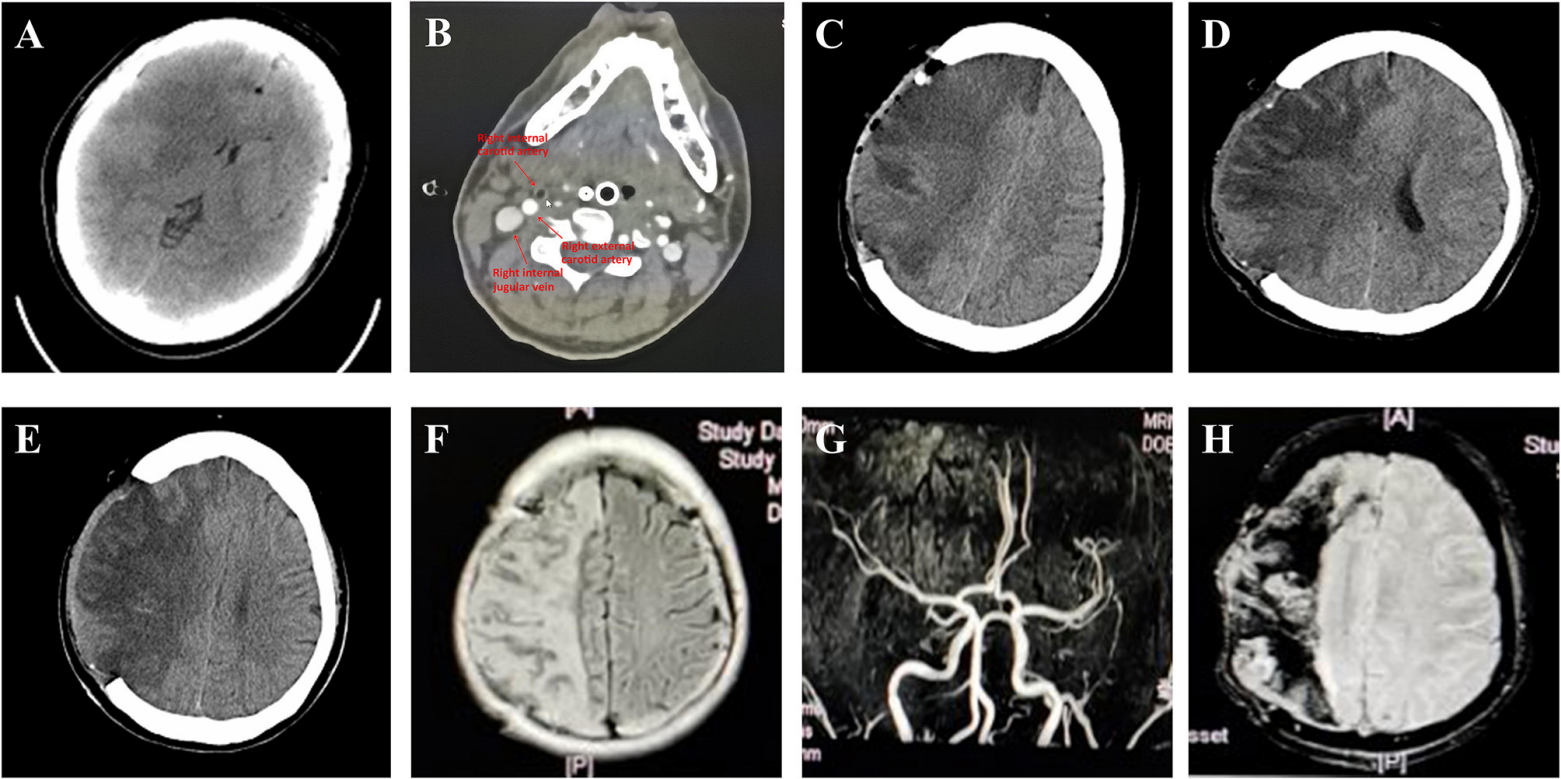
Imaging in different stages after cerebral infarction of this case. (A) Brain CT imaging of the patient shows massive cerebral infarction on the right frontal, temporal, and parietal lobes, and basal ganglia. (B) The neck CTA suggested that the right external carotid artery was occluded. ICA, ECA, or jugular vein are pointed out with arrows. (C) Post-surgery brain CT imaging of the patient. (D) Post-surgery Brain CT imaging of the patient 1 week post-surgery (2019-11-11). (E) Post-surgery Brain CT imaging of the patient 12 days post-surgery (2019-11-16). Images show improvement of edema, emorrhage absorbed. (F) The follow-up MRI-FLAIR performed 1 month after onset showed attenuated brain edema, and (G) MRA performed 2 months after onset showed vascular recanalization of intracranial area. (H) MRI-SWAN performed 2 months after onset showed the hemorrhage was gradually decreased with no other obvious hemorrhage.
